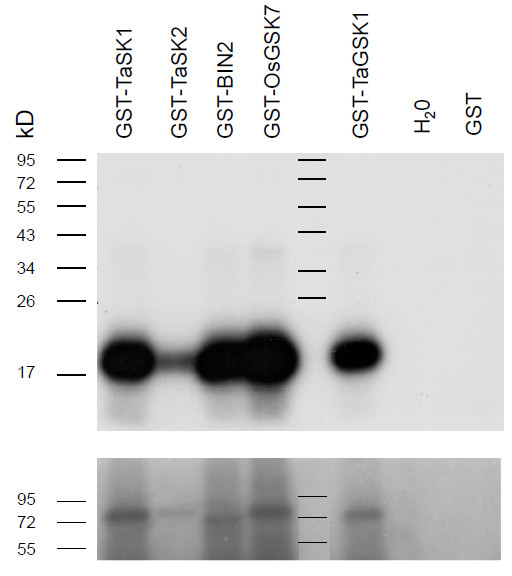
In vitro *kinase assays. Activity of the purified kinases was determined by *in vitro* kinase assays using γP^32^ATP. An equal volume of GST-TaSK1 (71,585 kDa), GST-TaSK2 (71,917 kDa) , GST-BIN2 (69,877 kDa), GST-OsGSK7 (72,174 kDa), GST-TaGSK1 (70.154 kDa), and GST (27,898 kDa) purified fusion proteins was used to test their phosphorylation activity on a myelin basic protein fragment (18,454 kDa). After kinase reaction, samples were loaded on a 12% SDS PAGE gel. After migration, the gel was directly exposed to an X-ray film for either 80 minutes (upper panel) or 24 hours (lower panel). OsGSK7 and BIN2 locus names are respectively RGAP: LOC_Os05g11730 and TAIR: AT4G18710, while the accession of TaGSK1 is GenBank: AF525086.
